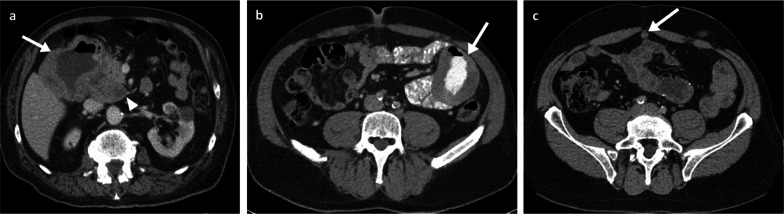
Duodenal and small bowel metastases. Portal phase contrast-enhanced CT axial (a) of an 80-year-old male patient with a large aneurysmal metastatic melanoma mass in the duodenum (arrow) and adjacent necrotic node (arrowhead). Oral and intravenous portal phase contrast-enhanced CT axial of a 57-year-old male patient with large jejunal melanoma metastasis (b: arrow) and anterior peritoneal nodule (c: arrow)
